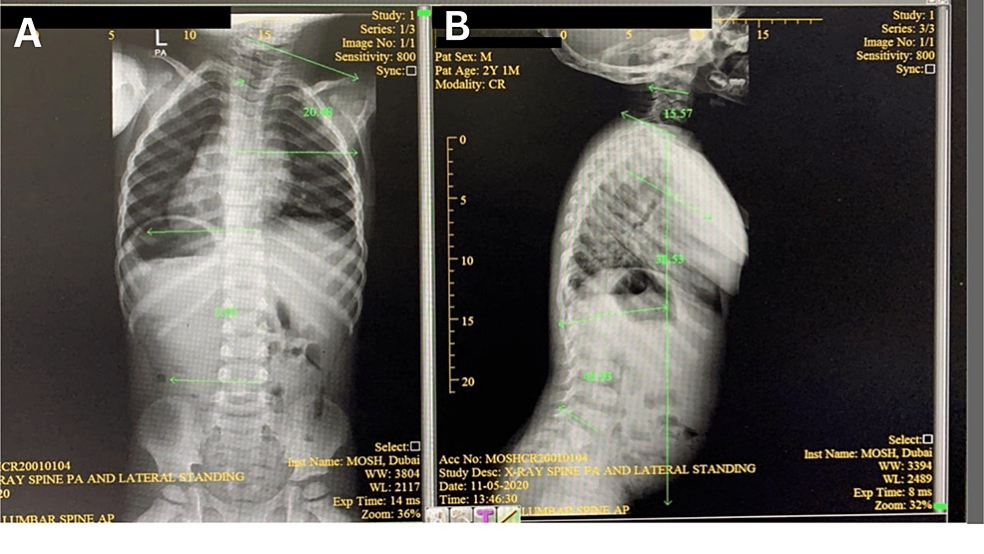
(A) Anterior and (B) lateral view of the X-ray images at three years old
